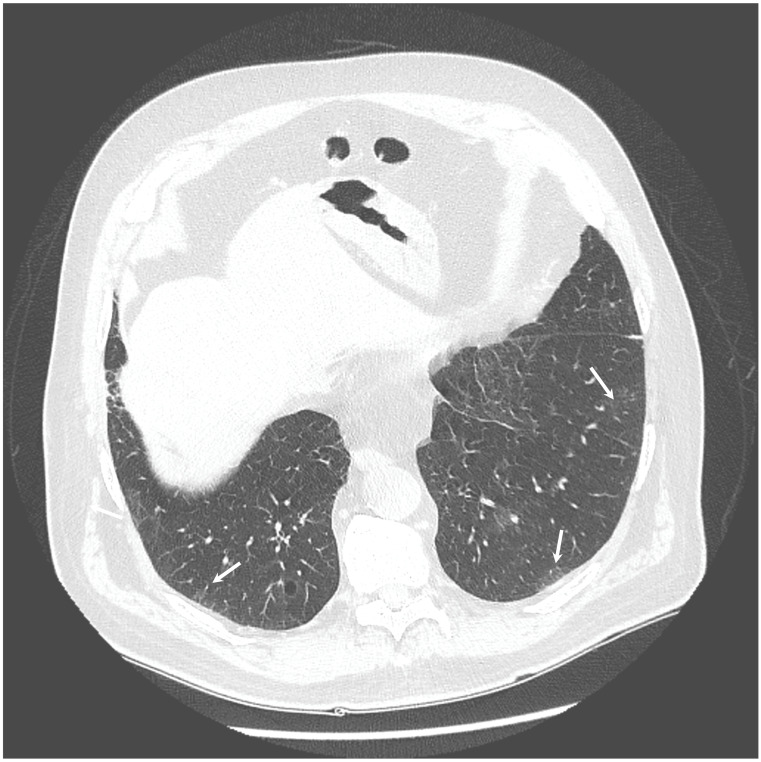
HRCT scan—Secretions accumulation and slight post-inflammatory changes (indicated by the white arrows).
